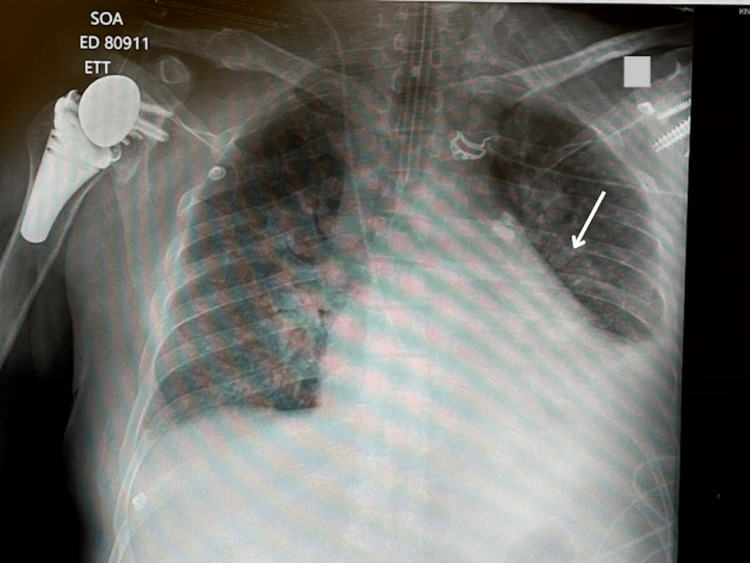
Portable X-ray of the chest demonstrating pleural effusions CXR: Chest x-ray. Arrow displays hazy densities, a fluid collection in the pleural space.
